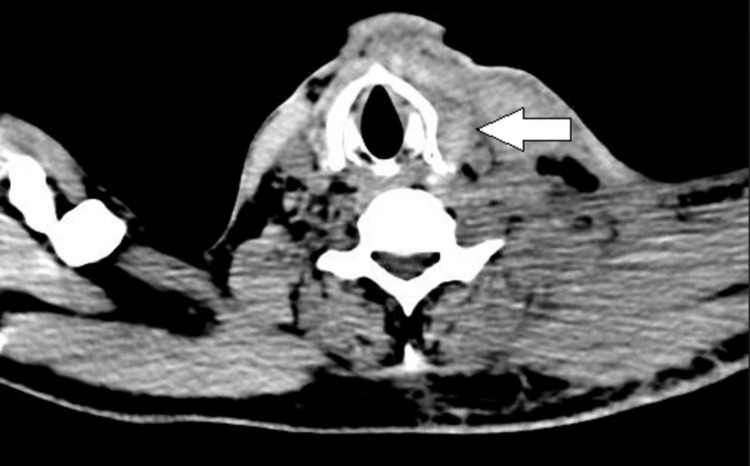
An axial cut of the computed tomography of the neck, showing compression of the laryngopharynx
